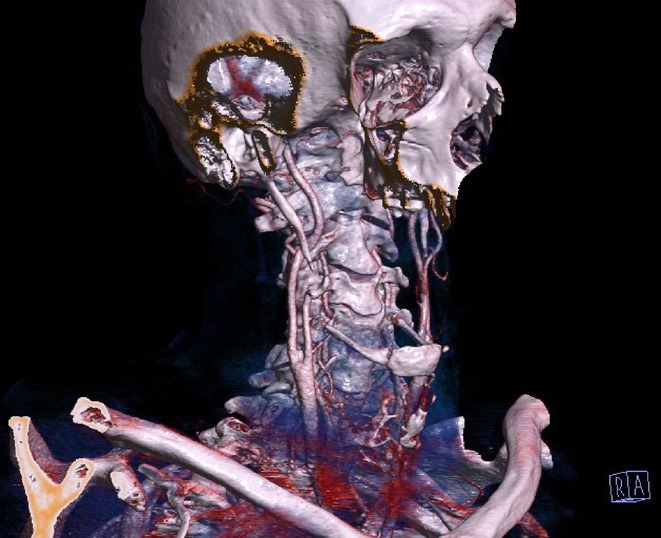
Image 1 3D reconstruction of CT angiography showing conflict of elongated styloid process and internal carotid artery on the right side.
